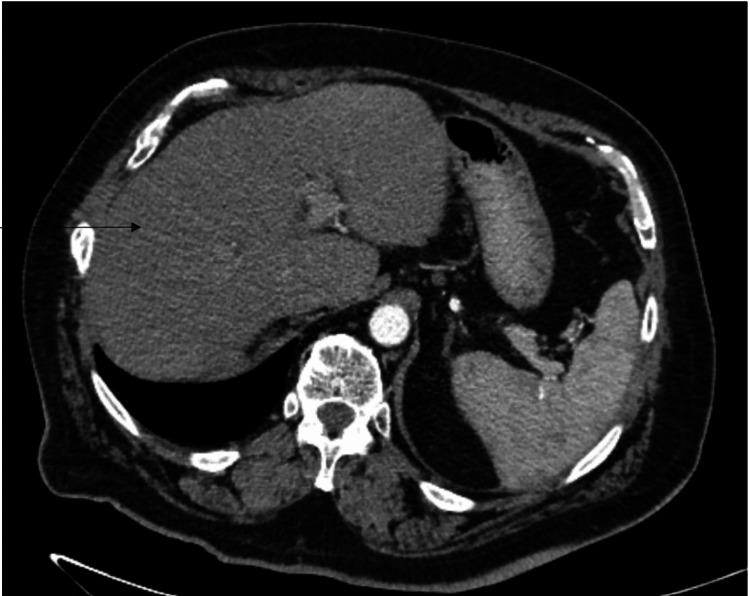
Stable liver appearance and absence of metastatic disease in chest, abdomen, and pelvis: follow-up CT imaging one year after treatment: arrow indicates no evidence of residual or recurrent disease
